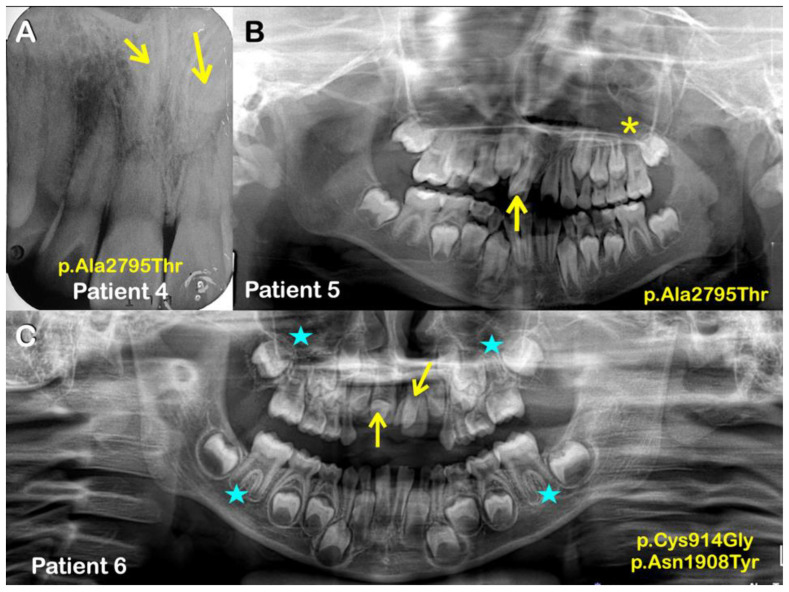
(A) Patient 4. Periapical radiograph showing double mesiodentes (inverted) (arrows). (B) Patient 5. Panoramic radiograph showing a mesiodens (arrow) and taurodontism (asterisk). (C) Patient 6. Panoramic radiograph showing double mesiodentes (arrows) and taurodontism (asterisks).
